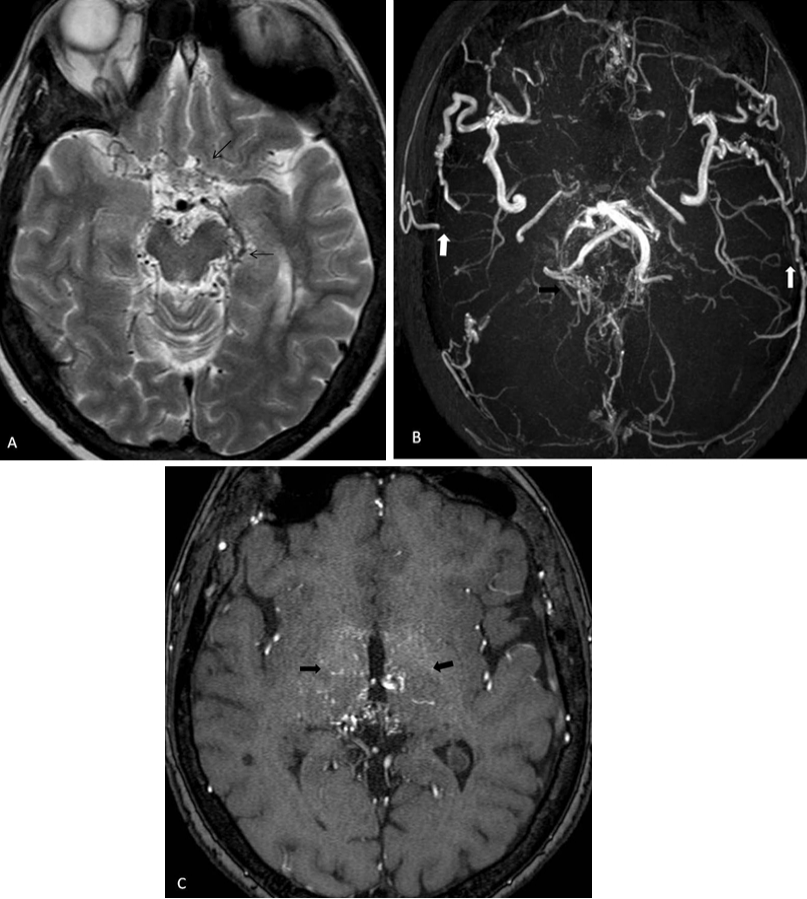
(a) Axial T2-weighted magnetic resonance (MR) image shows microvascular collaterals at the level of perimesencephalic cisterns and vascular supply to anterior cerebral artery and middle cerebral artery at the anterior circulation (arrows). (b) Axial MR angiography image reveals microangiopathic collaterals at the posterior circulation originating from basilar artery and its branches (black arrow). There was vascular supply from an external carotid artery by collateral development (white arrow) and bilateral internal carotid arteries occlusion at the petrous segment. (c) Axial MR angiography image demonstrates microangiopathic collaterals resembling puff of smoke (moyamoya vessels) at thalamus and basal ganglia (arrows).
